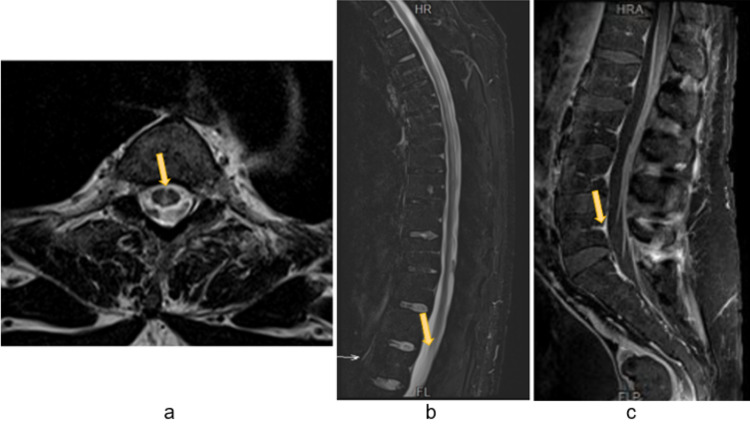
Spinal cord MRI - longitudinal T2-weighted hyperintensities in the dorsal columns of the spinal cord (arrows in panels a and b). Sagittal T1-weighted image showing pial conus medullaris and cauda equina gadolinium enhancement (arrow in panel c).
